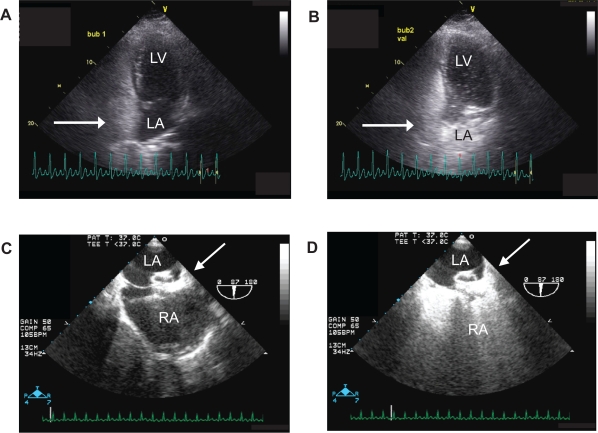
Panel A) TTE, apical four-chamber view. This image was obtained while the patient was breathing normally. Bubble contrast has opacified the right atrium (arrow). There is no evidence of bubble contrast in the left heart: left atrium and left ventricle are marked LA and LV, respectively. Prolonged imaging after contrast injection did not reveal late appearance of contrast in the left heart, indicating that a pulmonary shunt is not likely present (data not shown). Panel B) TTE, apical four-chamber view. This image was obtained during the Valsalva maneuver. Bubble contrast has opacified the right heart (arrow). Contrast has opacified the left atrium (LA) and a significant amount of contrast is visible in the left ventricle (LV): this contrast appeared within three cardiac cycles after contrast reached the right heart. Panel C) Intraprocedural TEE without bubble contrast, performed after the occlusion device was percutaneously deployed. The left atrium (LA) is visible at the top of the image, with the interatrial septum and the right atrium (RA) beneath. The echo-dense mass in the interatrial septum is the device (arrow). Panel D) Intraprocedural TEE with bubble contrast, performed after the occlusion device was percutaneously deployed. The view in this image is identical to that in Figure 3C with the exception of contrast. Opacification of the right atrium (RA) is visible in this image, with no evidence of passage of contrast across the device (arrow) into the left atrium (LA).
